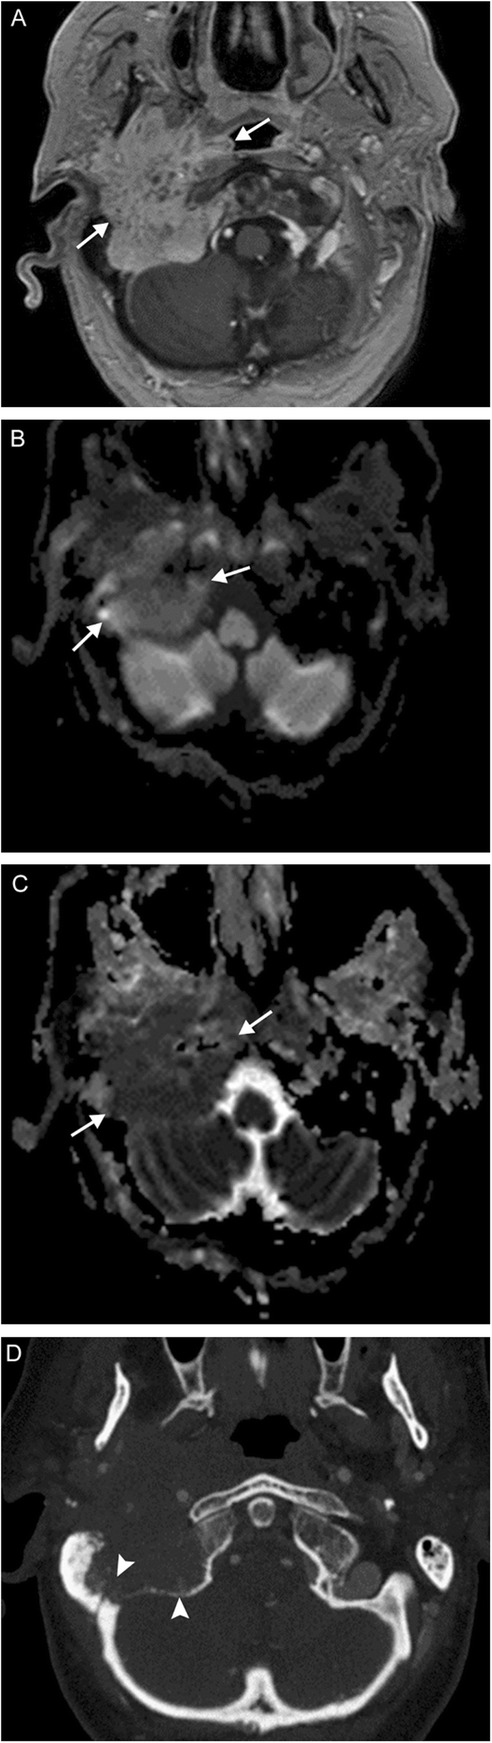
Skull base metastasis. A 63-year-old woman with several weeks of right-sided ear infection and hoarseness, followed by sudden onset right facial droop. Axial post-contrast T1-weighted (a) and DWI/ADC (b, c) MR images reveal a large enhancing infiltrative lesion with diffusion restriction at the skull base (arrows). Axial CTA image (d) demonstrates osseous destruction and obliteration of the right internal jugular vein (arrowheads). This was found to be metastatic adenocarcinoma of unknown primary
